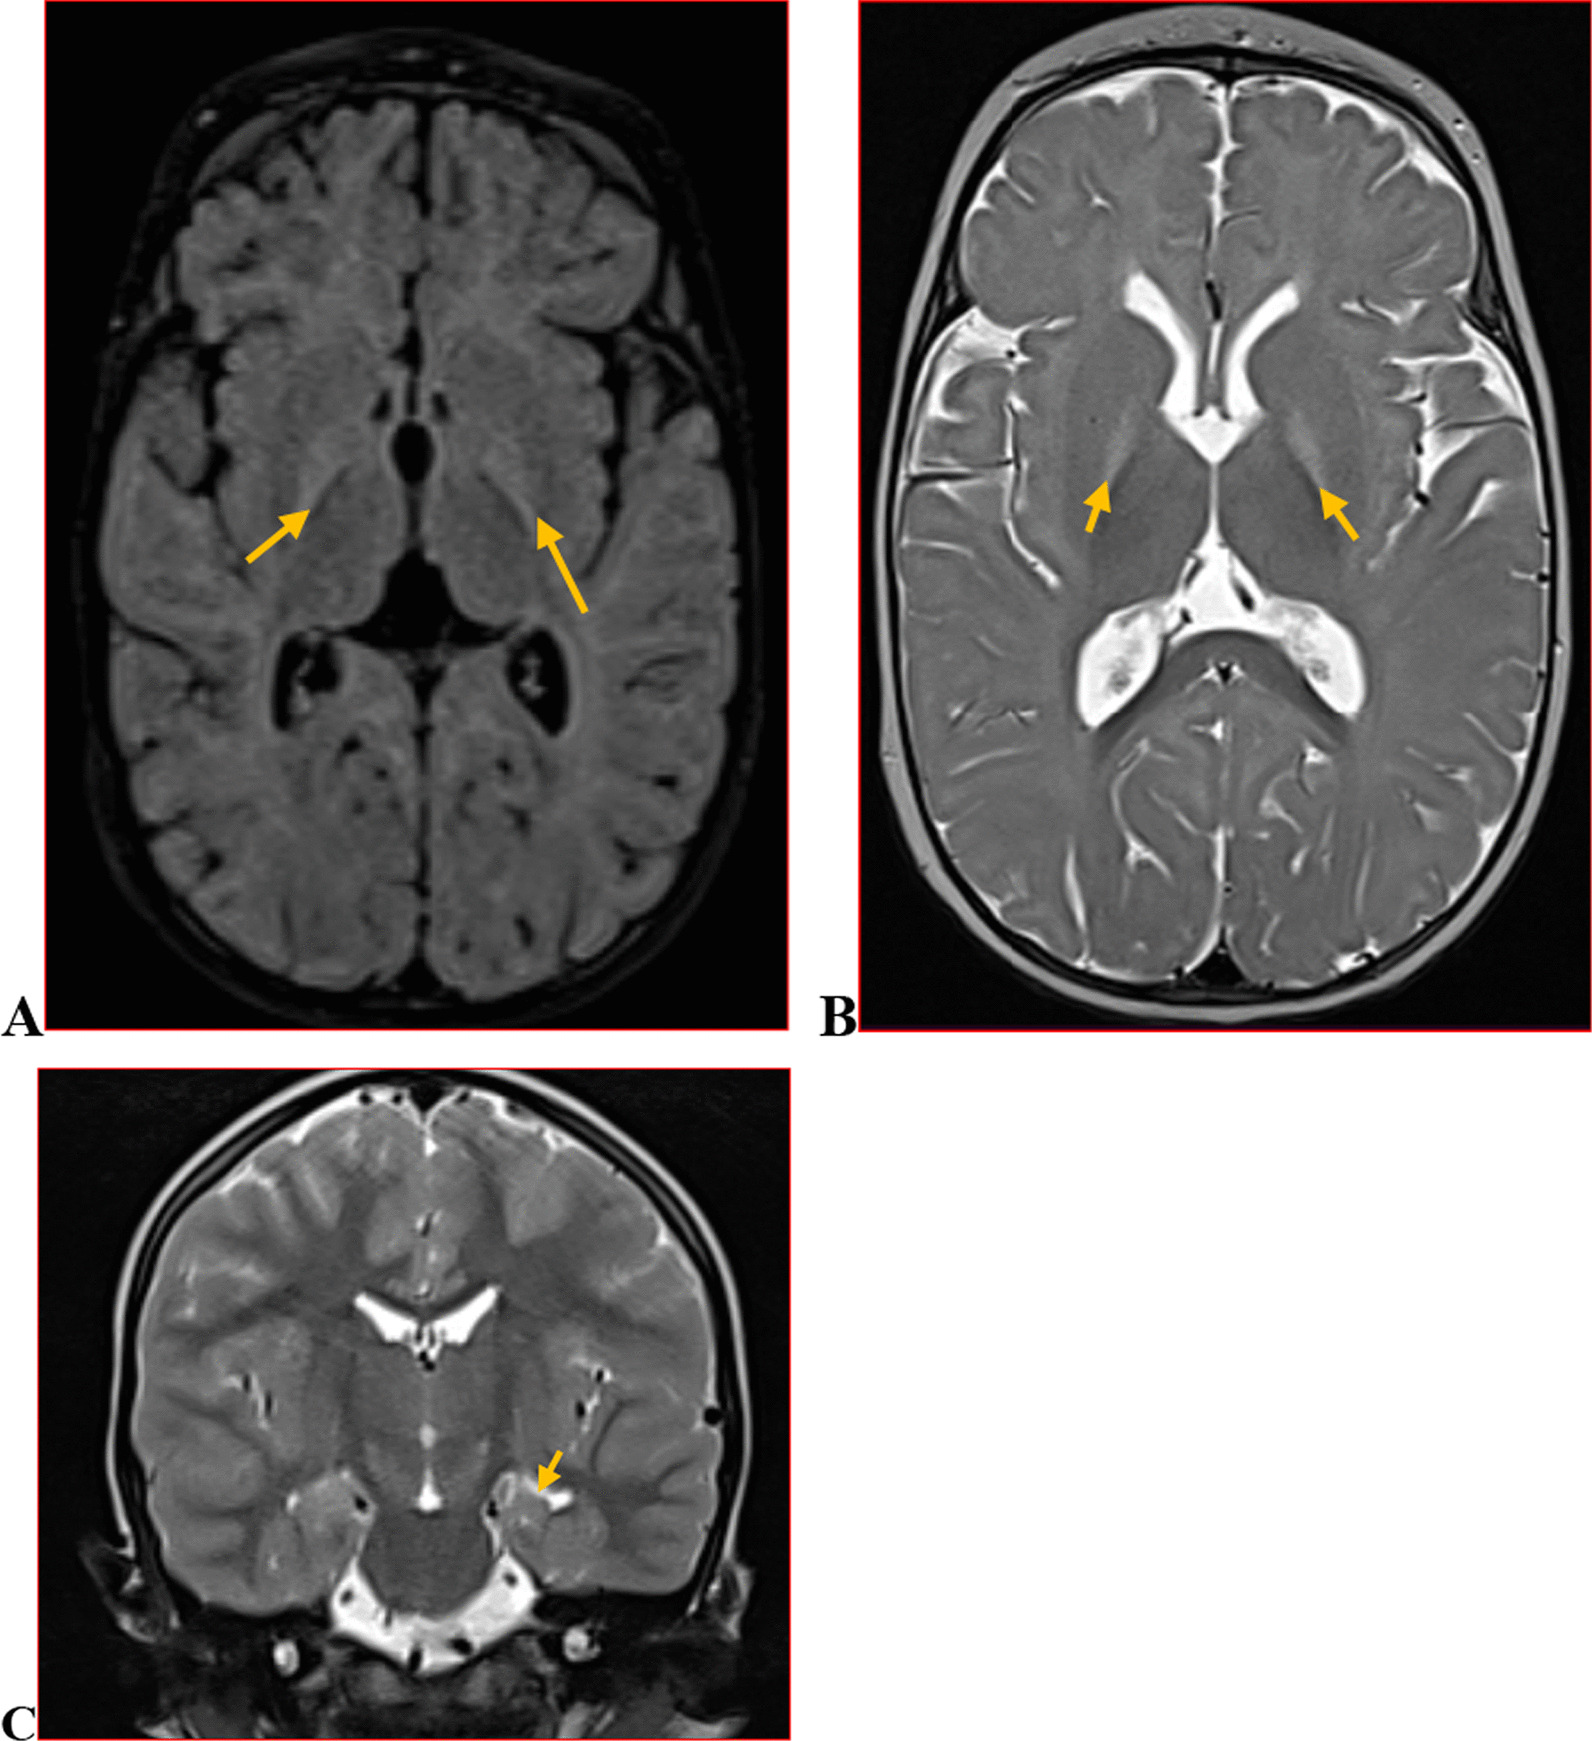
Symmetric globus pallidus T2/FLAIR hyperintensity(arrows) (A and B) in a 6 year old female with history of infantile jaundice. Findings are in keeping with Kernicterus.Additionally,the patient had left hippocampal atrophy depicted in C (arrow)
